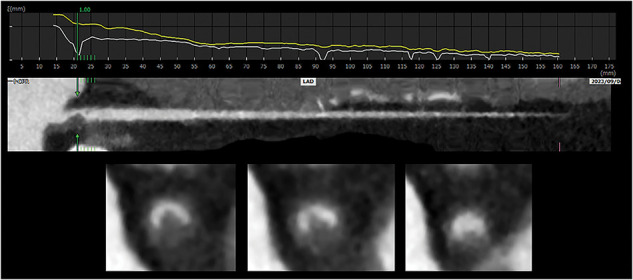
CCTA before surgery. CCTA showed 75% stenosis of the true lumen of the LMT due to compression by the false lumen. CCTA, coronary CTA; LAD, left anterior descending artery; LMT, left main trunk
